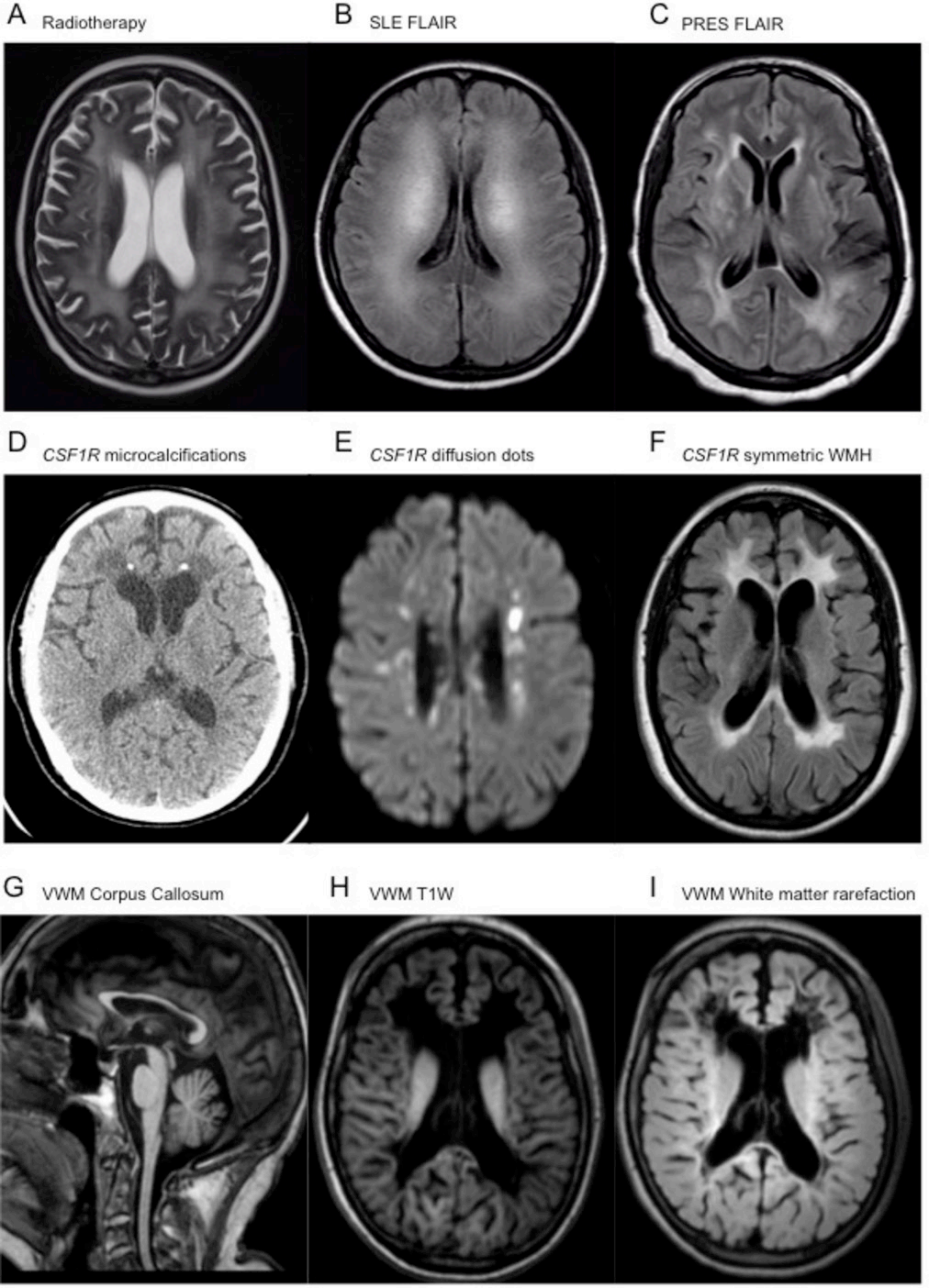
Axial T2-weighted MRI of radiotherapy changes (A), as well as axial FLAIR MRI of cerebral Systemic Lupus Eythematosus (SLE) (B) and PRES (C) demonstrating symmetrical and confluent periventricular and subcortical white matter signal hyperintensity (WMH). Axial non-contrast CT demonstrating subcortical volume loss, punctate calcification and confluent hypodensity adjacent to the frontal horns of the lateral ventricles (D). Axial B1000 DWI and FLAIR MRI acquisitions showing DWI avid punctate lesions (E) as well as confluent periventricular hyperintense signal changes (F) in *CSF1R* leukodystrophy. MRI sagittal T1W acquisition showing diffuse thinning of the corpus callosum (G), as well as axial T1W (H) and FLAIR (I) sequences demonstrating confluent rarefaction of the subcortical and periventricular white matter adjacent to the frontal horns and ventricular trigones of the lateral ventricles in VWM disease. FLAIR, fluid-attenuated inversion recovery; PRES, posterior reversible leukoencephalopathy syndrome; T1W, T1-weighted; VWM, vanishing white matter.
